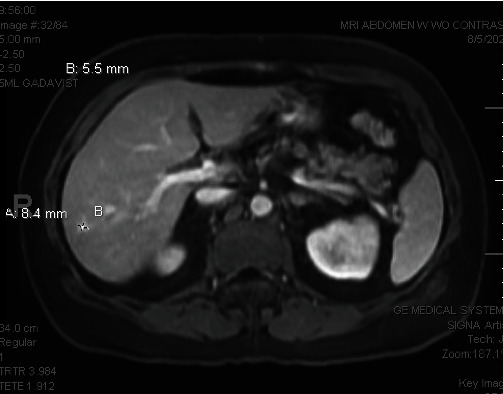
MRI of the abdomen without contrast. Image provided courtesy of Orlando Health.
